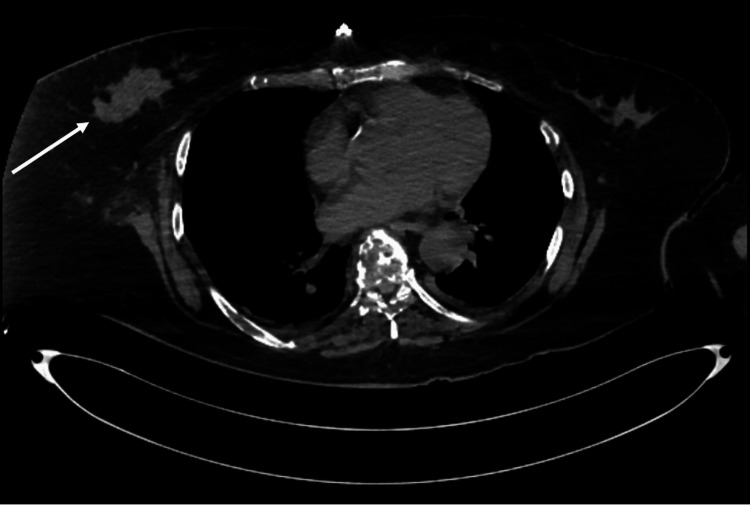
Computed Tomography (CT) Showing a Right Breast Lesion Axial chest CT demonstrating an irregular solid lesion in the right breast with irregular morphology and heterogeneous density, suspicious for malignancy (white arrow).
